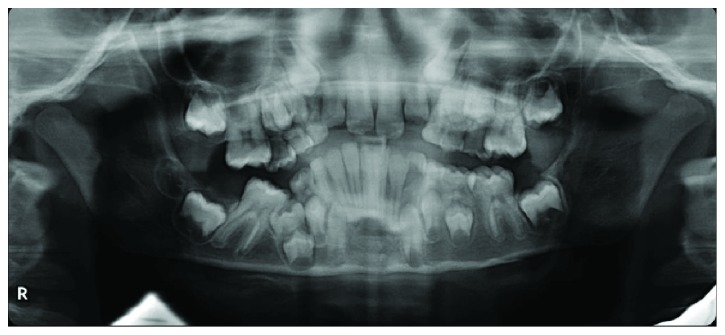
Orthopantomogram of the case at first examination. The second deciduous molar appeared severely infraoccluded. The first molar (4.6) was severely inclined mesially.
